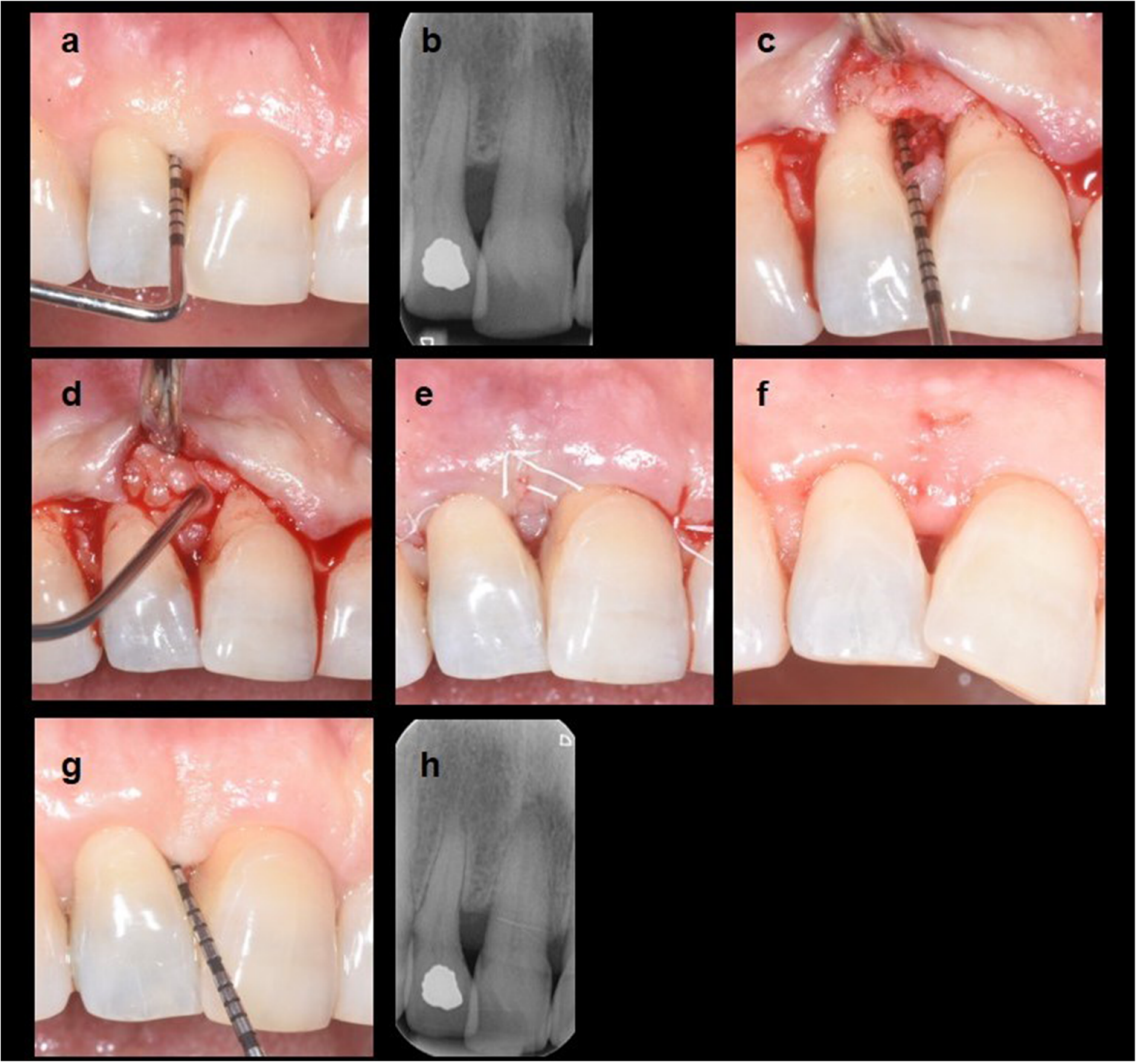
Treatment of a supraosseous defect with SFA+EMD. (a) supraosseous pocket (PD=8mm) at the distal aspect of the upper right second incisor, (b) radiographic aspect of the supraosseous lesion at pre-surgery, (c) clinical aspect immediately after access with a buccal SFA and surgical debridement, (d) EMD application after root conditioning with EDTA, (e) clinical aspect immediately after suturing, (f) complete wound closure with absence of fibrin line at the incision margins as observed at 2 weeks after surgery, (g) 12-month pocket closure, and (h) radiographical aspect at 12 months
